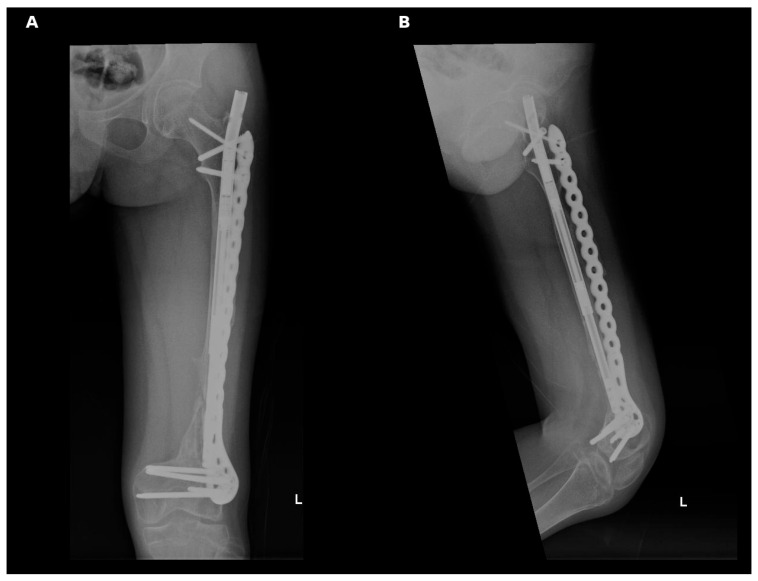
Final follow-up radiographs demonstrating the end result after bone transport and subsequent docking-site reconstruction. (A) Anteroposterior radiograph including the adjacent hip and knee joints. (B) Lateral radiograph including the adjacent joints.
